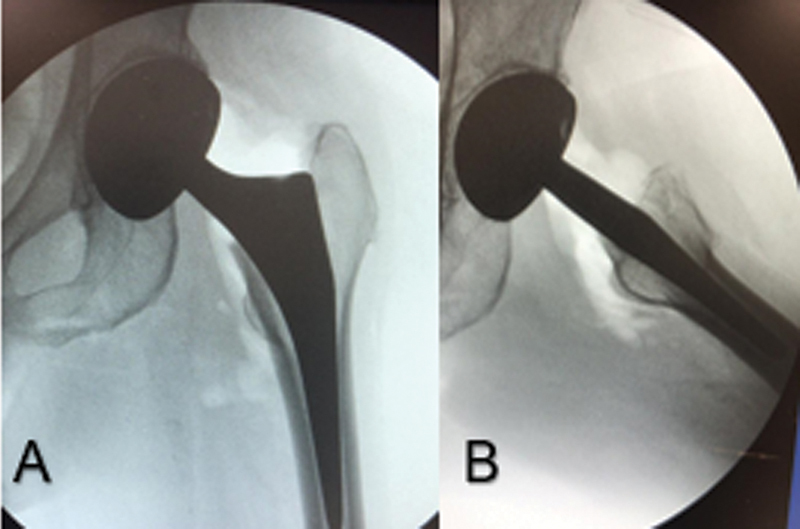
Radiographic control in the immediate postoperative period showing reduction of components and concentricity of the metallic head (A) in AP and profile (B).
